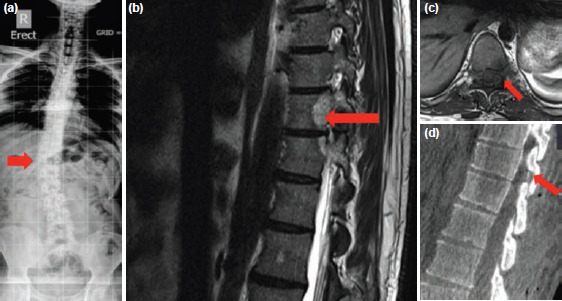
(a) Radiograph full length anteroposterior view showing the scoliosis with convexity to the right. (b) A well-defined lytic lesion seen on MRI sagittal cut involving the superior articular process of the D11 vertebra. (c) MRI axial cut showing lesion superior articular process of D11. (d) Intra-operative image showing adequacy of extirpation using the navigation probe in sagittal image.
